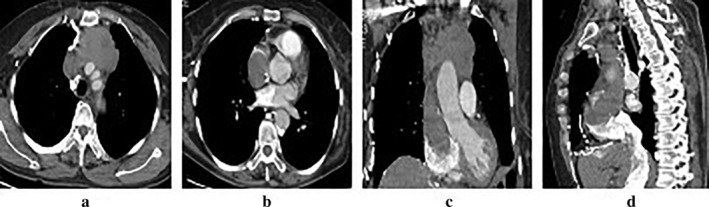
Computed tomographic (CT) scan with intravenous contrast administration. (a–b) Axial views: A large irregular anterior mediastinal mass with heterogeneous enhancement was seen to obstruct the SVC and had extended into the right atrium. (c–d) Coronal and sagittal views: the mass located in the anterior mediastinum encased the mediastinal great vessels, and infiltrated into the right atrium.
